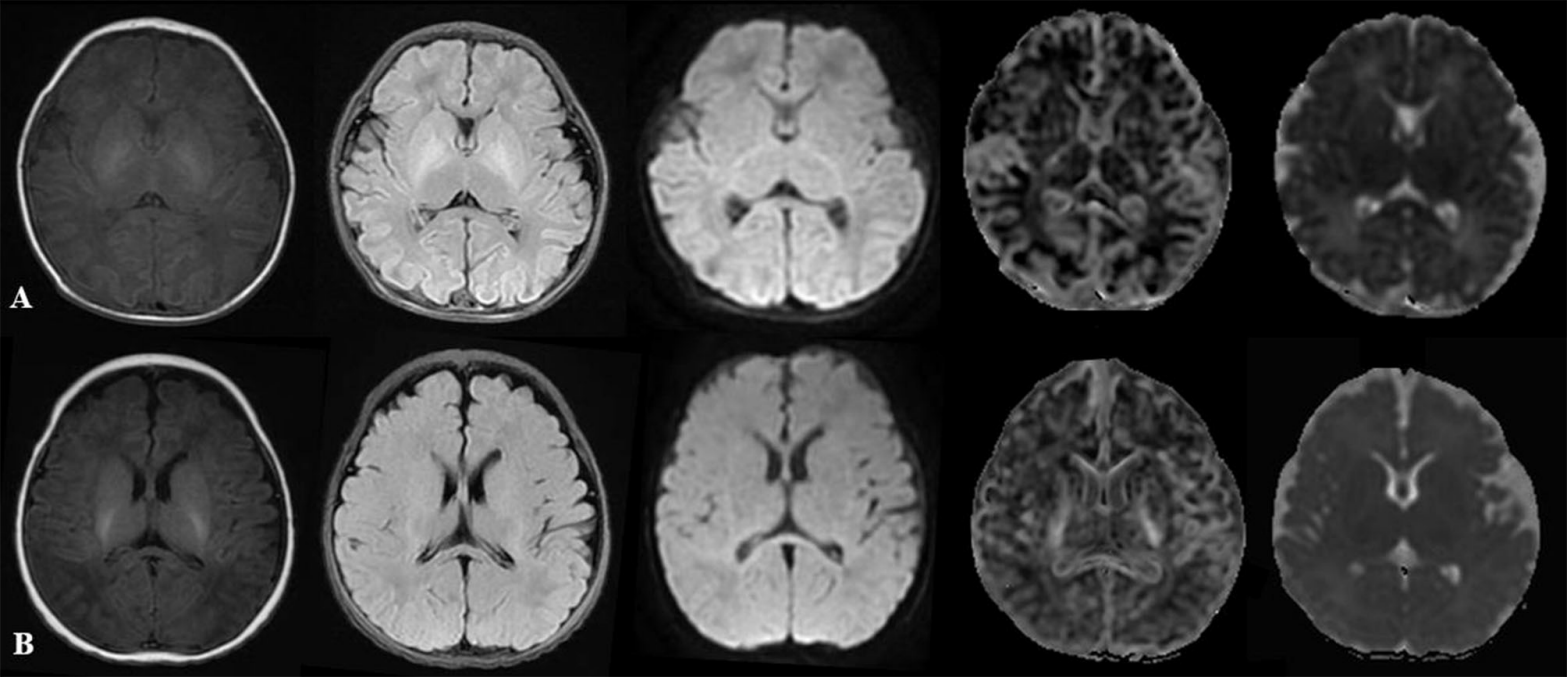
Comparison of multiparameter images in different PMAs. (A) represented: male, GA 30 weeks, PMA 42 weeks. (B) represented: male, GA 37 weeks, PMA 43 weeks. From left to right: T1WI flair, T2WI flair, DWI, MK, and MD maps. DWI, diffusion-weighted imaging; PMA, postmenstrual age; GA, gestational age; MK, mean kurtosis; MD, mean diffusivity.
